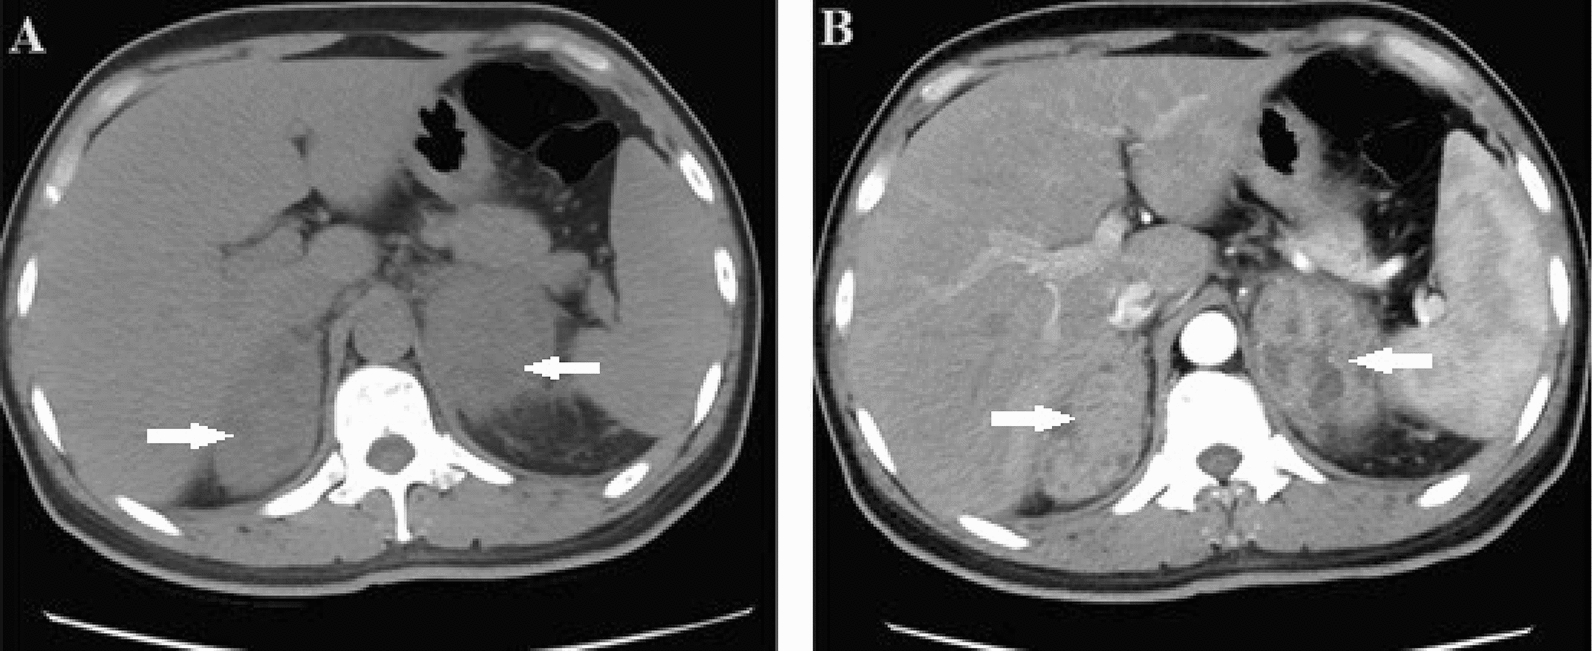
A Transverse view of unenhanced computed tomography (CT) showed hypodense and heterogeneous solid lesions adjacent to both adrenal glands. B Transverse view of Enhanced CT showed moderate and heterogeneous enhancement of masses
